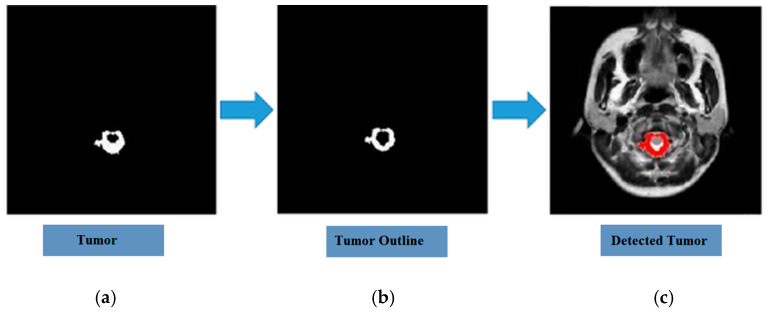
Progression of tumor delineation: (a) isolated pathological region; (b) boundary contour overlay on the anatomical scan; (c) holistic detection within the complete MRI field of view.
